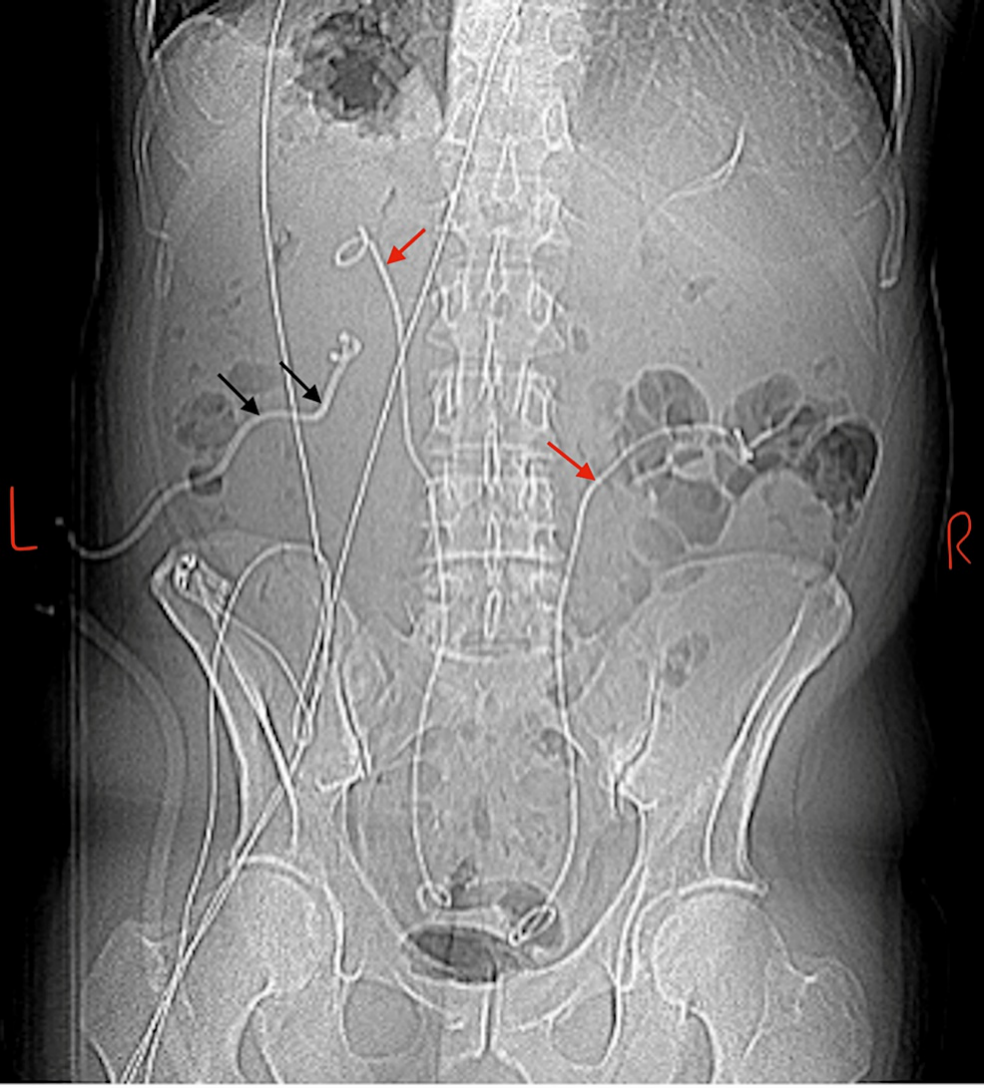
Computed tomography scan Scout image showing bilateral ureteric stents (in red arrows) and left nephrostomy (in black arrows).
